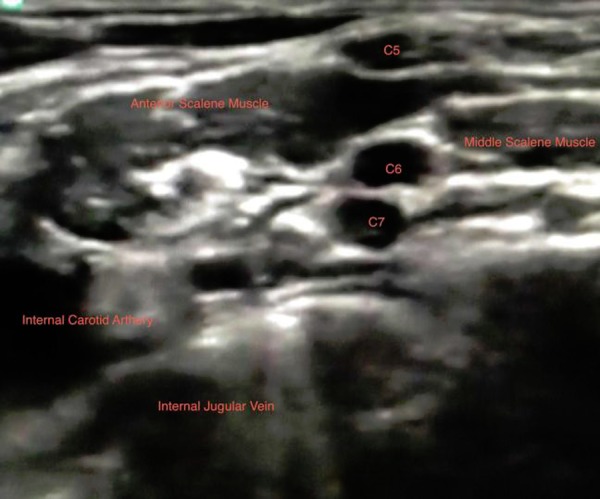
Sonographic anatomyof the brachial interscalenic plexus.
